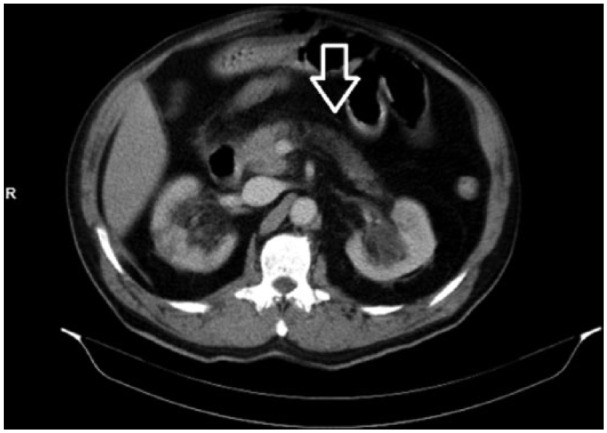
Axial computed tomography image of Case 1. Loss of lobulation of the pancreas and small amounts of peripancreatic fluid are observed.
